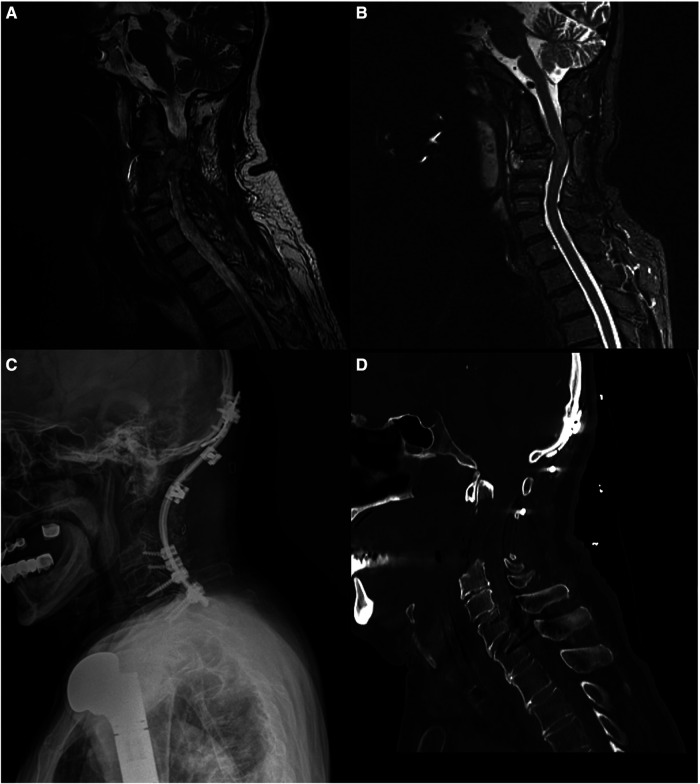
(A) A pre-operative MRI scan showed a lesion located in the C3 vertebra. (B) MRI scan of the recurrent lesion prior to the third surgery (C) Cervical x-ray after occipitocervical stabilization surgery. (D) Cervical computed tomography (CT) scan after tumor resection and copectomies of C2, C3, and C4 vertebrae.
